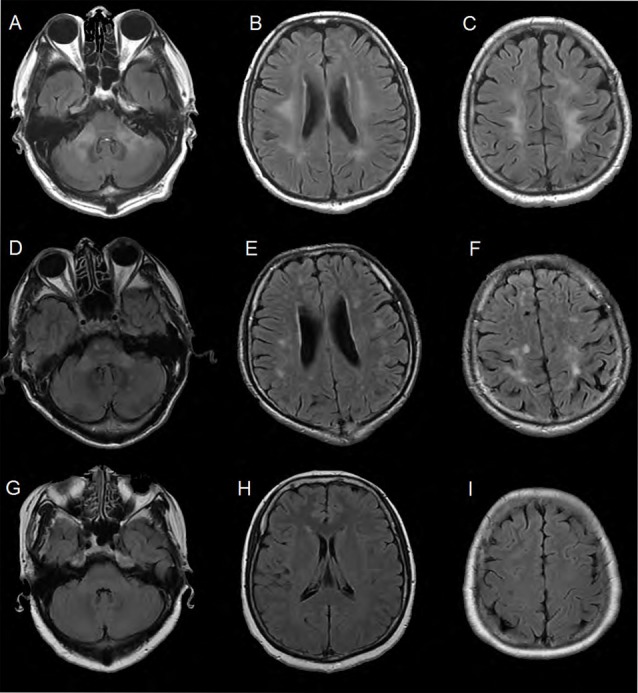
Brain MRI of the family members. (A–C) Were FLAIR images of the proband. There were symmetric confluent long T2 signals in mid-cerebellar peduncles, periventricular areas and centrum semi-oval respectively. (D–F) Were obtained from the proband’s younger brother (III-12). The images showed patchy hyper-intensities in the same or adjacent planes, which was milder than the proband’s scan. (G–I) Were images of the proband’s elder sister (III-10). There were no white matter (WM) lesions in the same areas.
